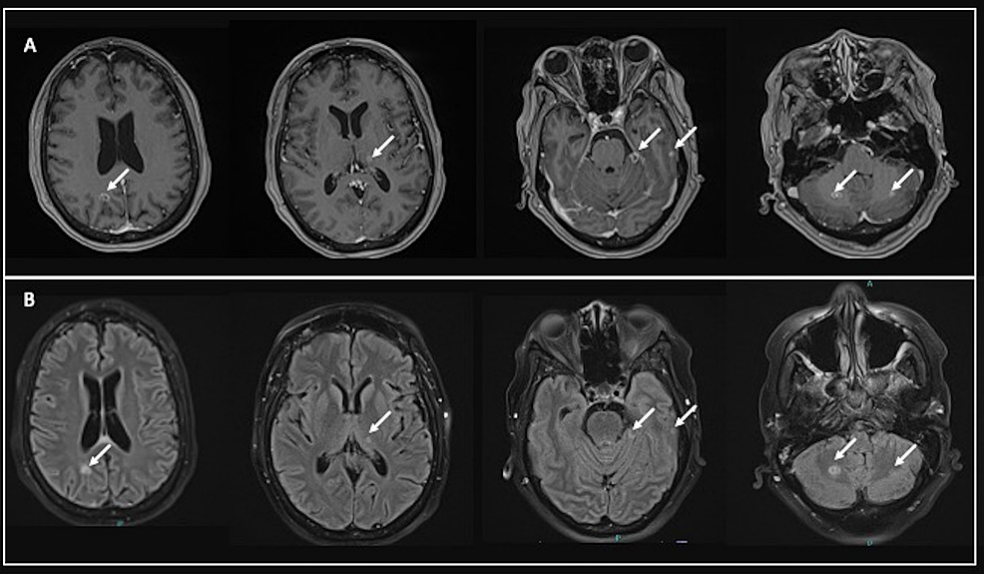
MRI of the brain with and without contrast. Panel A shows post-contrast axial T1 3D MPRAGE sequences demonstrating ring-enhancing lesions (arrows) in both supratentorial and infratentorial locations. Panel B shows axial T2 FLAIR sequences demonstrating hyperintense lesions (arrows) corresponding to the contrast-enhancing lesions in both supratentorial and infratentorial locations. MRI: magnetic resonance imaging; FLAIR: fluid-attenuated inversion recovery; MPRAGE: magnetization-prepared rapid acquisition gradient-echo
